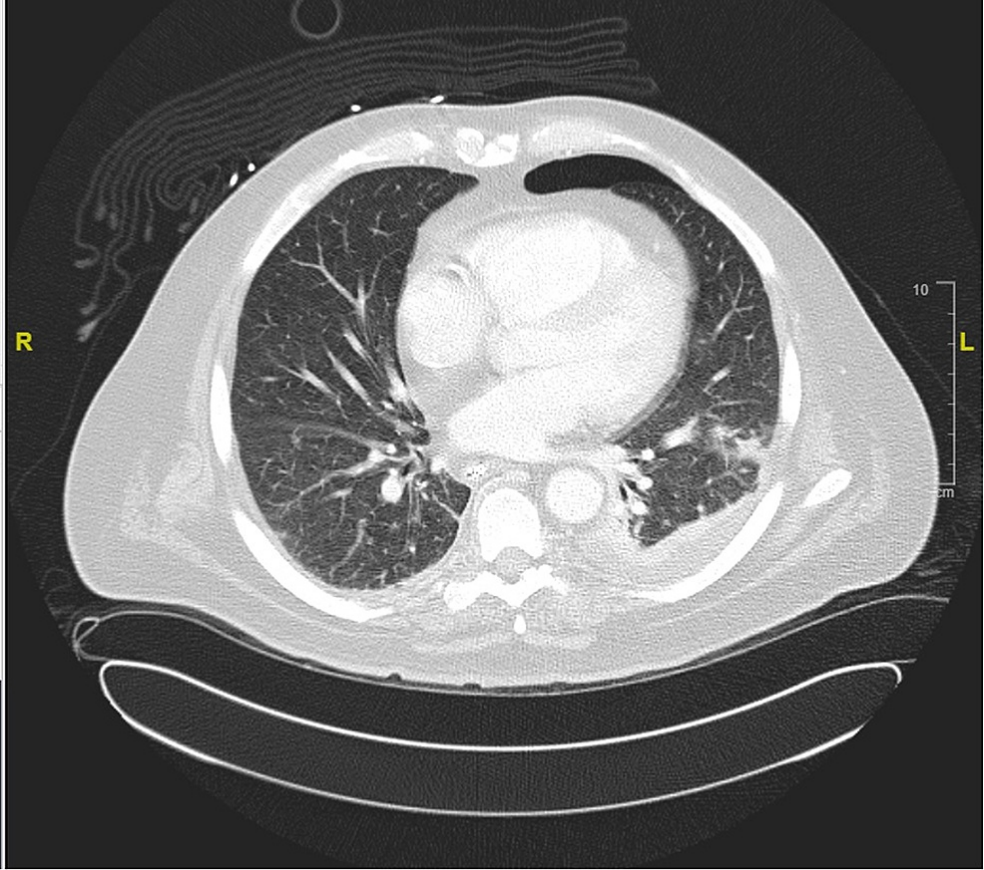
CT of the thorax showing regions of pulmonary consolidation in the left lung
